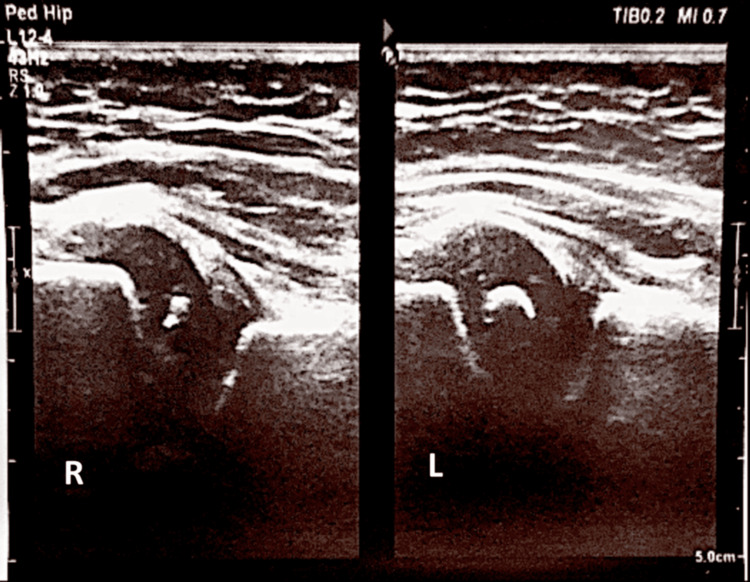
Follow-up hip ultrasound showing satisfactory reduction and appearance of the femoral head ossification nucleus. R: right. L: left
